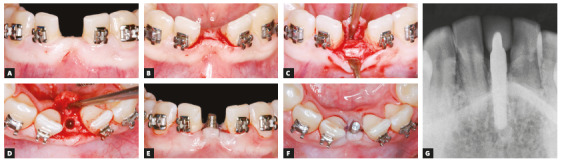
Sequence of placement between teeth #31 and #41 of one-piece osseointegrated implant. A) Anesthesia; B) incision; C) flap reflection; D) bone drilling; E, F) suture; G) periapical radiograph to evaluate implant position.
